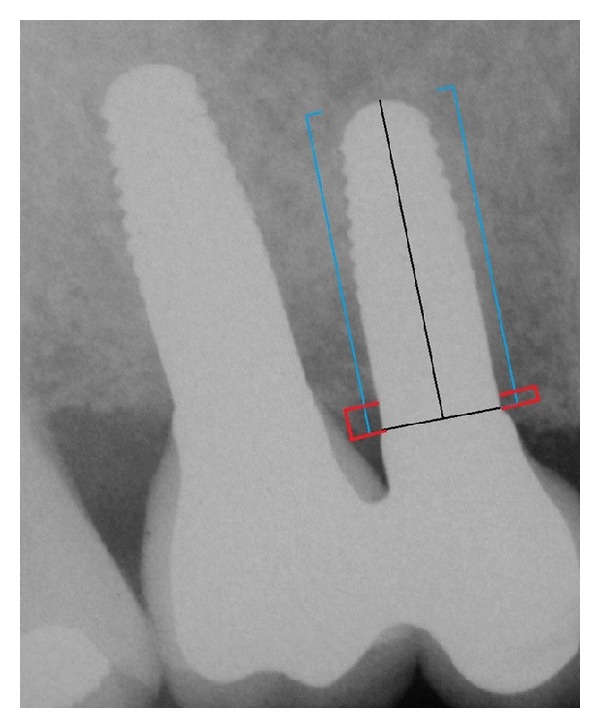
X-ray picture 12 months after surgery. The blue lines indicate the implant length; the red lines indicate the periimplant bone levels.
